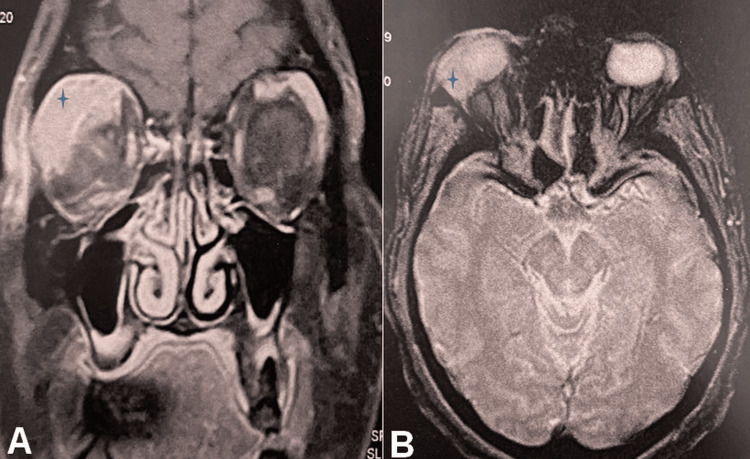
Coronal (A) and axial (B) MRI images showing a mass arising from the right lacrimal gland (star).
